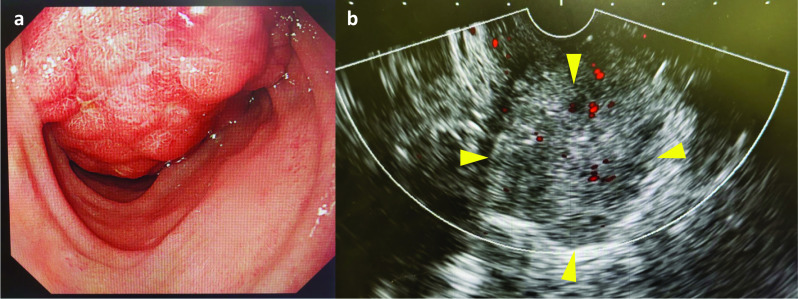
a Upper gastrointestinal endoscopy. A large papillary tumor (yellow arrowhead) occupying the second part of the duodenum. b Endoscopic ultrasonography. A hypoechoic ampullary mass (yellow arrowhead) showing no invasion of the surrounding organs such as the pancreas or bile ducts
